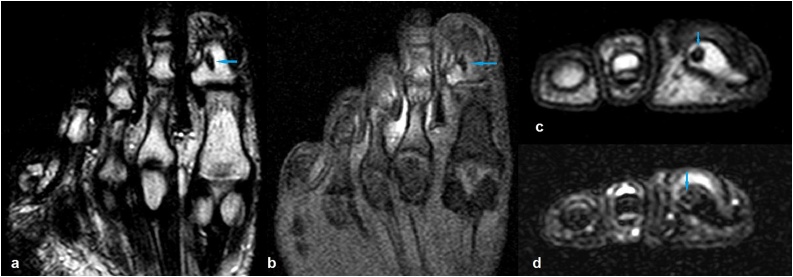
Coronal T1- and fat suppressed T2-weighted (a, b) and axial T1- and fat suppressed T2-weighted (c, d) magnetic resonance images demonstrating an osteoid osteoma in the distal phalanx of the hallux.
